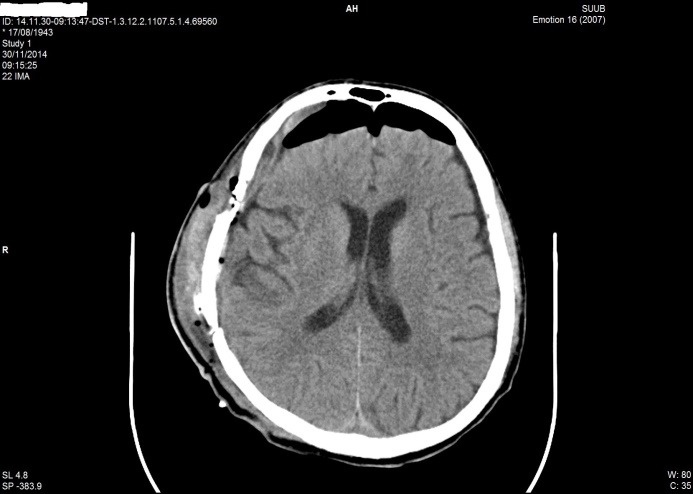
Postoperative CT aspect in patient B.I. of 71 years old; evacuated subdural haematoma, re-expansion brain, ventricular system on the median line
